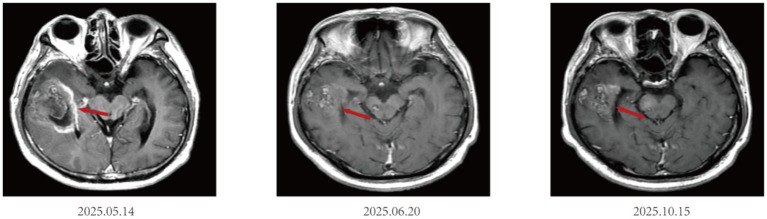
Brain MRI T1WI + C evolution during combination therapy for small cell lung cancer with brain metastases.
